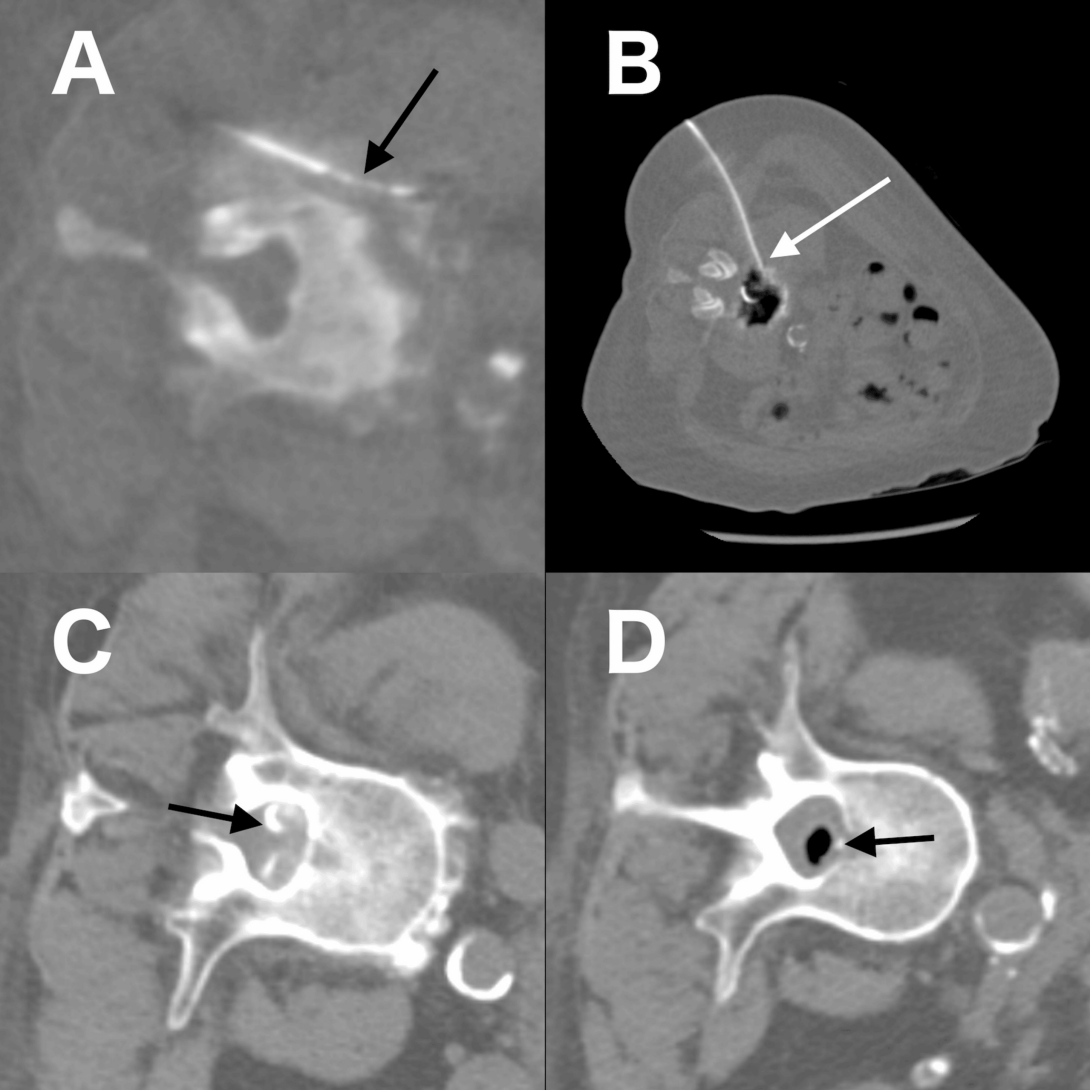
CT-guided intervention findings on day 15 A: Biopsy needle at the L4 perivertebral calcification (black arrow); B: Drainage tube placement (white arrow) in the L3/4 intervertebral disc space; C: Contrast injection through the drainage tube demonstrating partial epidural enhancement at the L3 level (black arrow); D: Epidural air at the L2 level after lavage, indicating epidural decompression and partial communication between the L2/3 disc and epidural spaces (black arrow)
